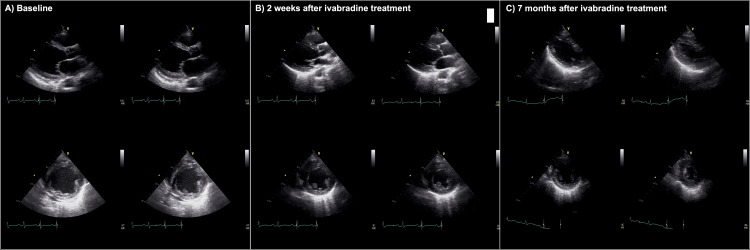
Transthoracic echocardiography before and after ivabradine The upper row presents the parasternal long axis view, and the lower row presents the parasternal short axis view. (A) Baseline. (B) Two weeks following ivabradine treatment. (C) Seven months following ivabradine treatment.
